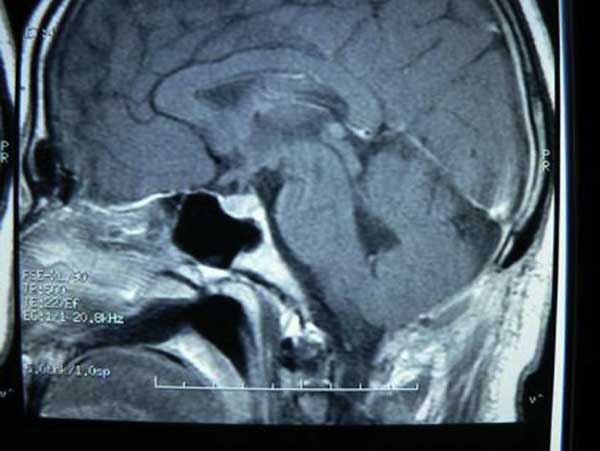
Post-operative magnetic resonance image of brain revealing no residual tumor and restoration of the 4^th^ ventricular contour.
